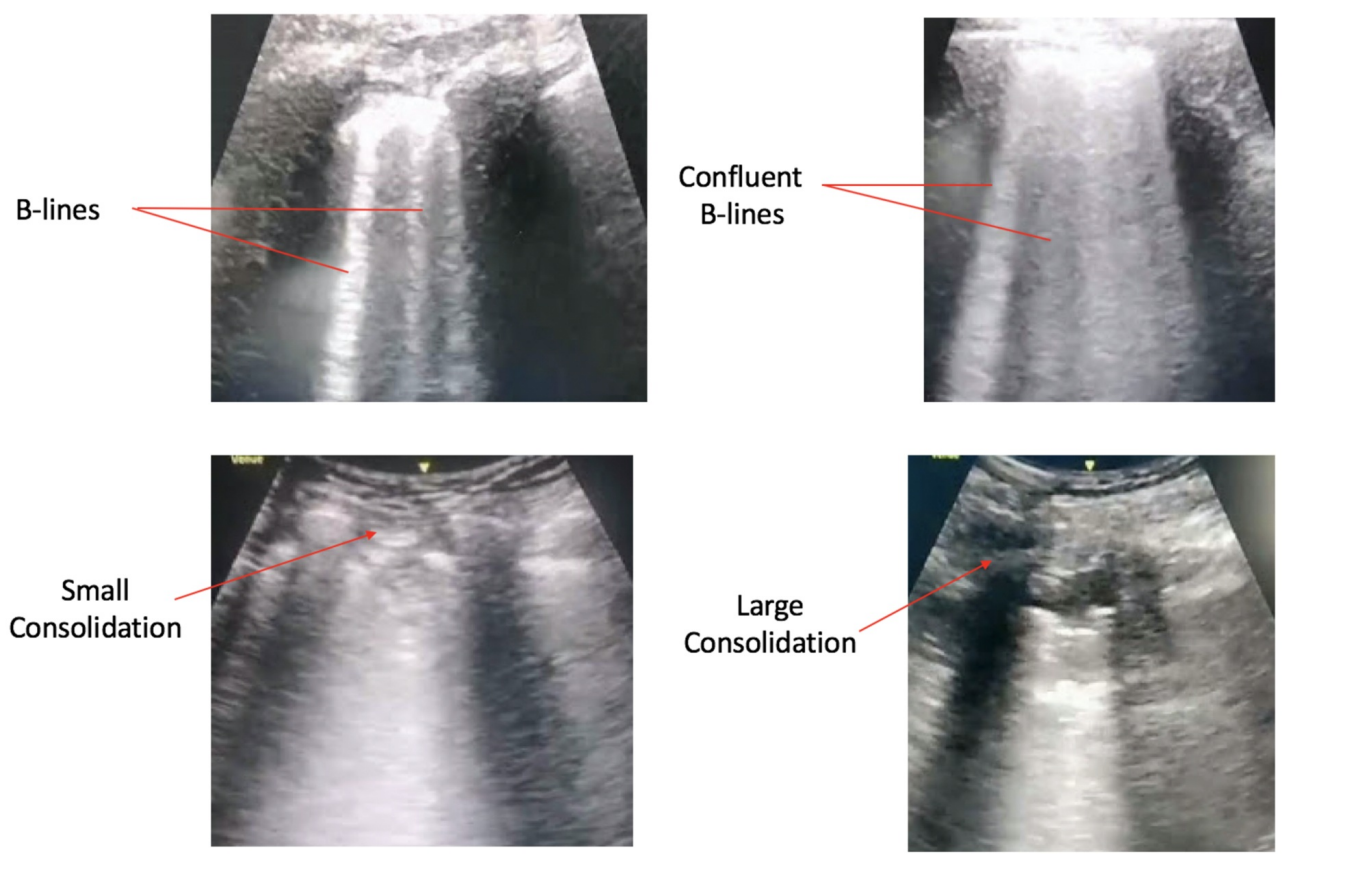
Lung ultrasound findings in patients with COVID-19
